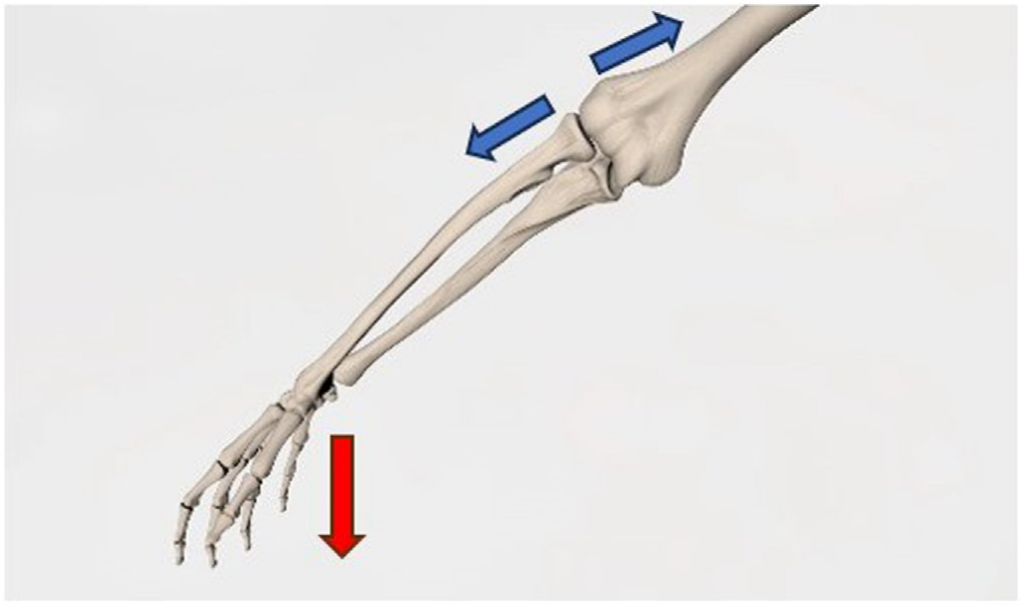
Anatomical representation of the US stress test. The weight on the wrist emphasizes the joint widening in pathological conditions, whereby the extensor muscles are unable to provide sufficient resistance to the applied load. *US*, ultrasound.
